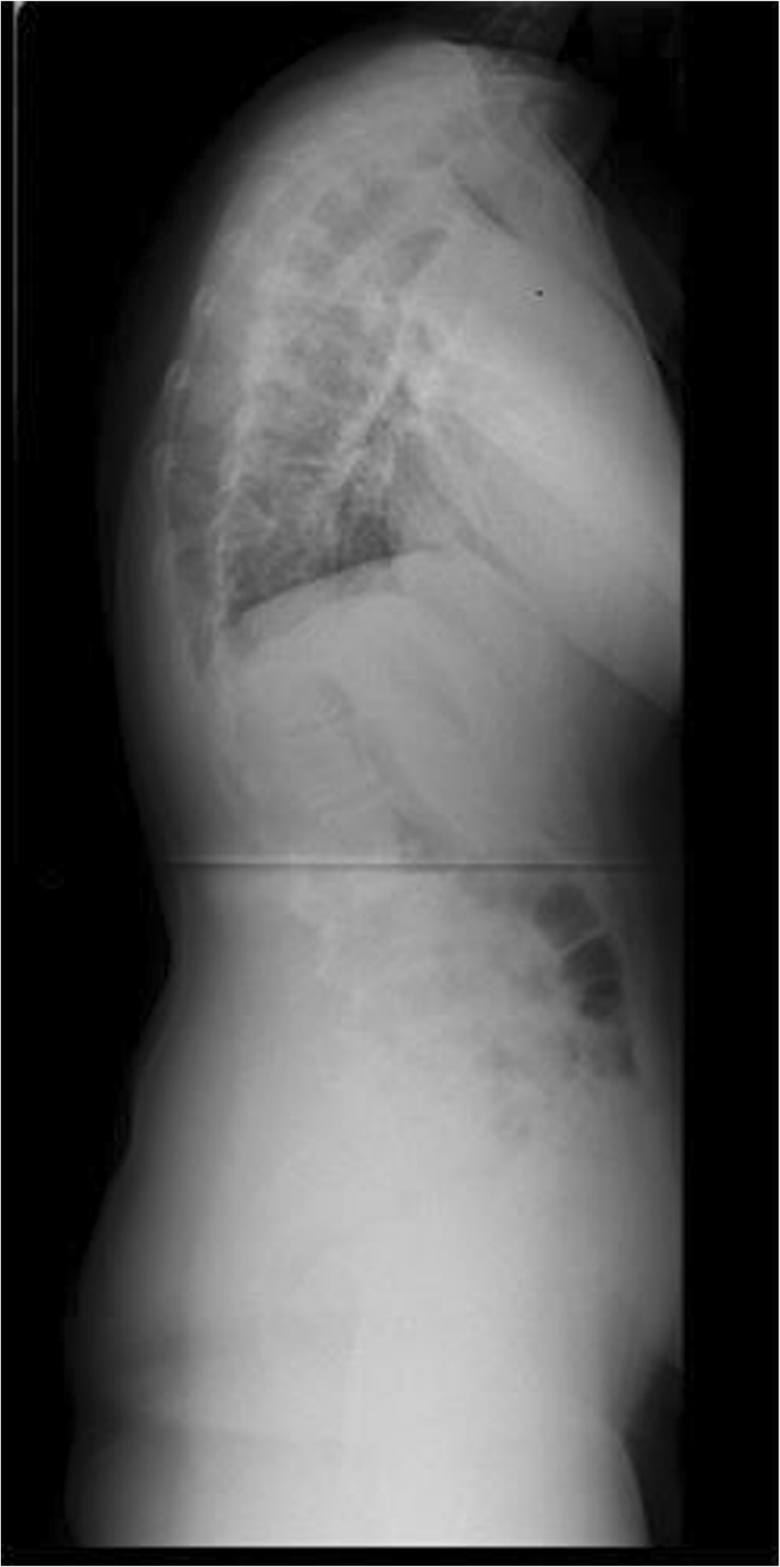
Pre-operative radiograph of 15 year-old male with Marfan syndrome.
